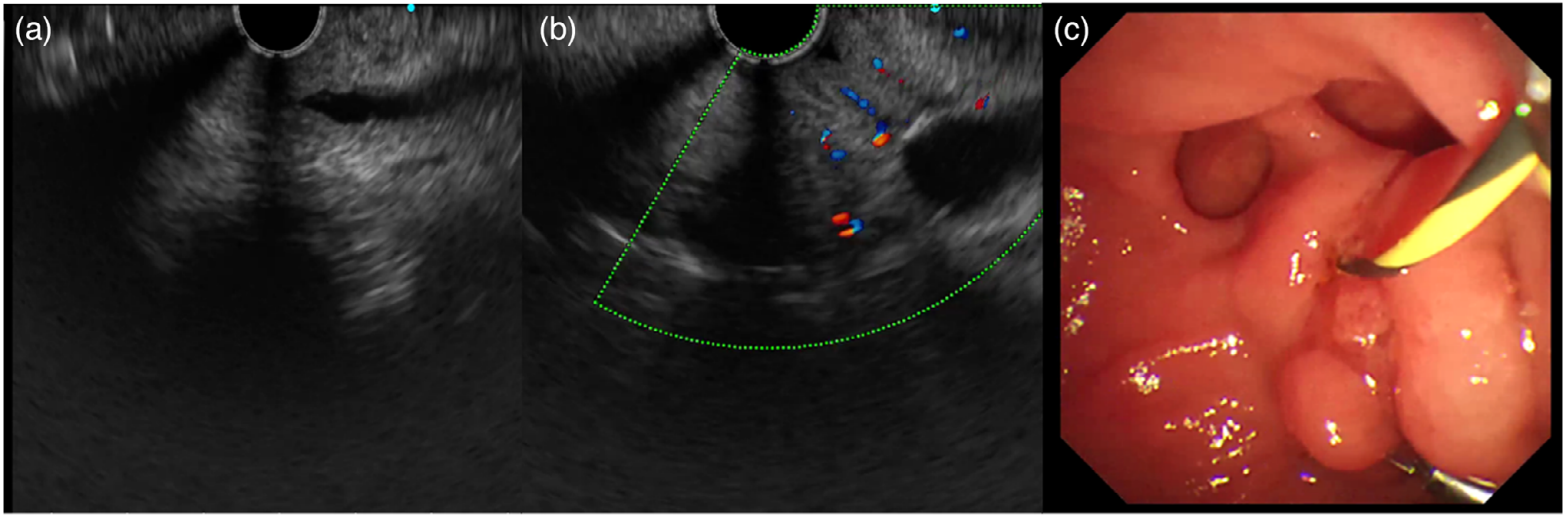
(a) Common bile duct and papilla 2 months post‐papillectomy showed no residual lesion on endoscopic ultrasound. (b) Examination of the common bile duct during endoscopic ultrasound (higher magnification). (c) Papilla 2 months post‐papillectomy.
